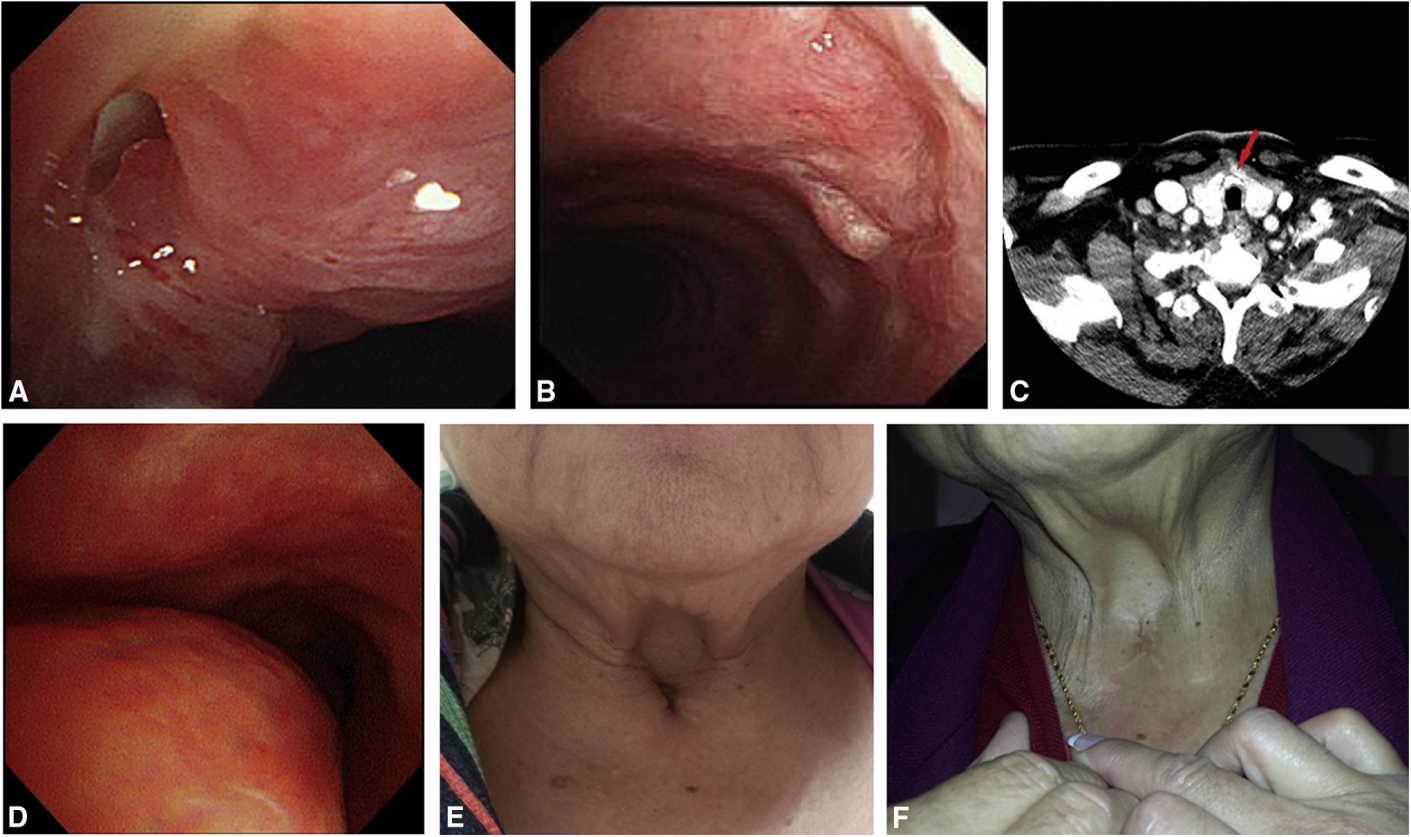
Postoperative assessment. A and B, Endoscopic zoom in and zoom out perspective views of the graft at 1 month postoperatively in the second patient, showing the nail graft well integrated with the native cartilage rings and covered with respiratory epithelium. C, Neck CT of the fourth patient shows calcification of the nail graft (*red arrow*) and no impending tracheal obstruction at 3 months postoperatively. D, Preoperative bronchoscopy of the first patient reveals bulging of the membranous portion of the trachea. E, The first patient initially had tracheomalacia and retraction of the skin over the frontal part of the neck. F, At the most recent follow-up, the cosmetic appearance of the neck and airway symptoms have improved after the reconstruction.
